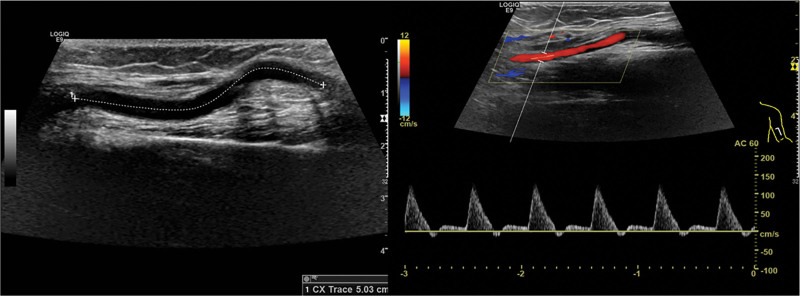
Postoperative follow-up ultrasonography. The self-grafted blood artery was curved and patency.
